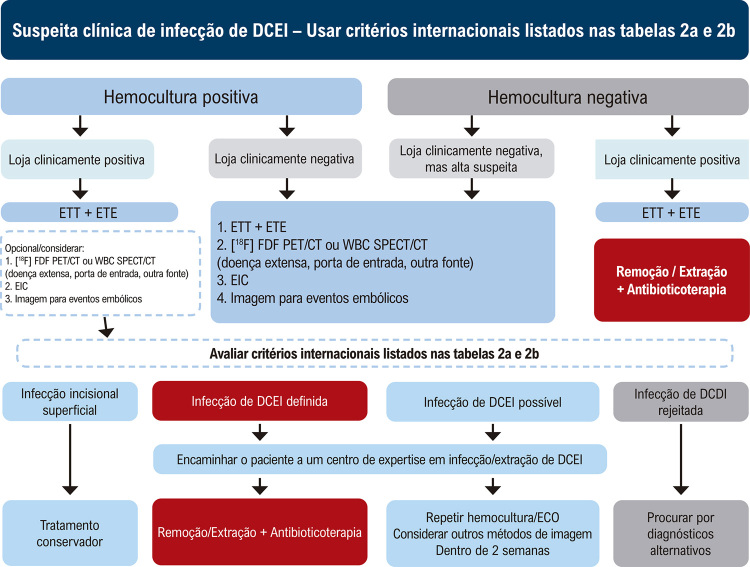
Fluxograma para diagnóstico e do tratamento de infecção de DCEI. DCEI: dispositivo cardíaco eletrônico implantável; ECO: ecocardiograma; ETT: ecocardiograma transtorácico; ETE: ecocardiograma transesofágico; FDG: fluordeoxiglicose; PET/CT: tomografia computadorizada por emissão de pósitrons; SPECT/CT WBC: tomografia computadorizada por emissão de fóton único (single photon emission computed tomography) com leucócitos marcados.
